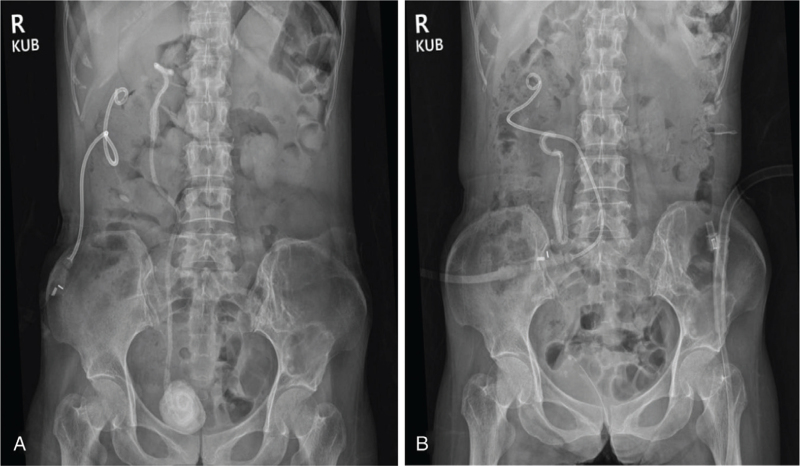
(A) X-ray Kidney-ureter-bladder (KUB) after percutaneous catheter drainage of right perinephric abscess. This revealed a double-J stent (DJS) with a large bladder stone formed in the lower coil. (B) On the 12th hospital day, a KUB scout image showed a DJS remnant adhered to the right proximal ureter and renal pelvis after endoscopic vesicolitholapaxy. DJS = double-J stent, KUB = kidney-ureter-bladder.
